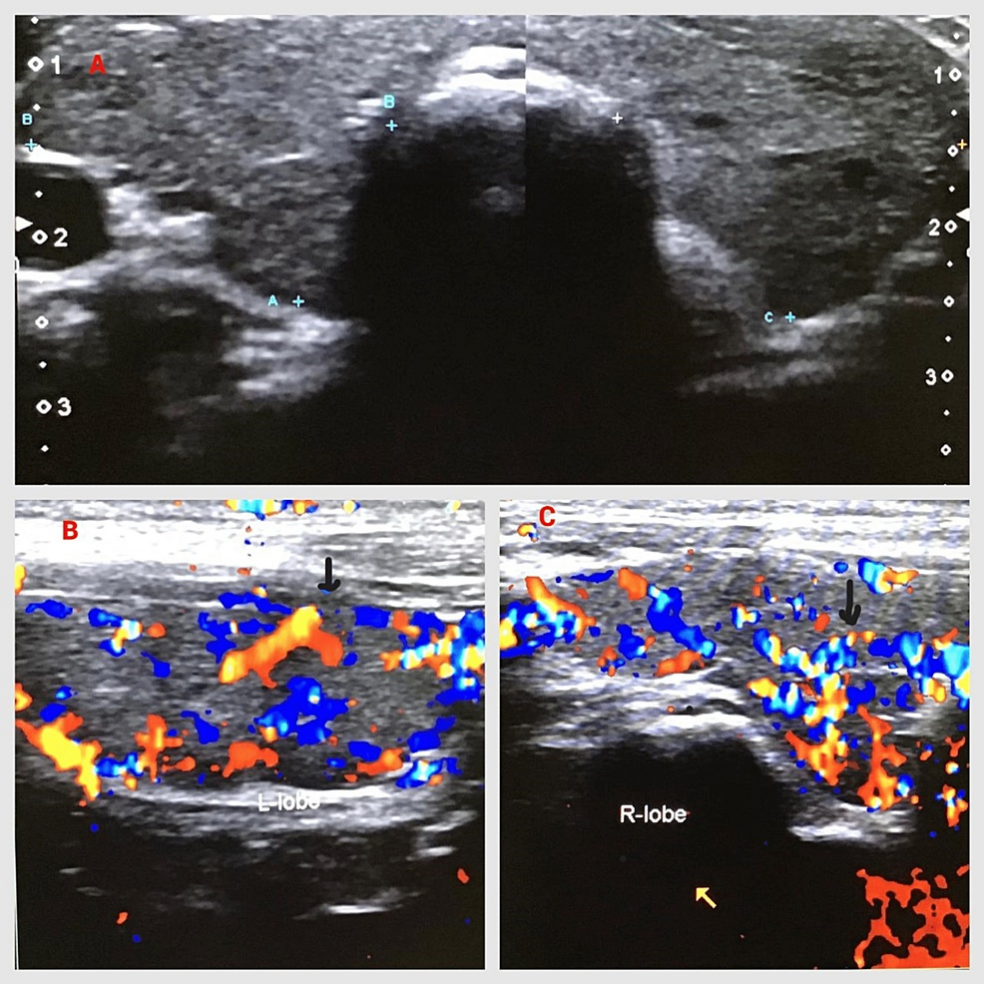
Ultrasound scan neck of the thyroid gland with the Doppler effect. A: Thyroid goiter with diffusely enlarged lobes. B, C: Left and right thyroid lobes with increased vascularity, suggestive of thyroiditis. Black arrows point to the higher vascular areas.
